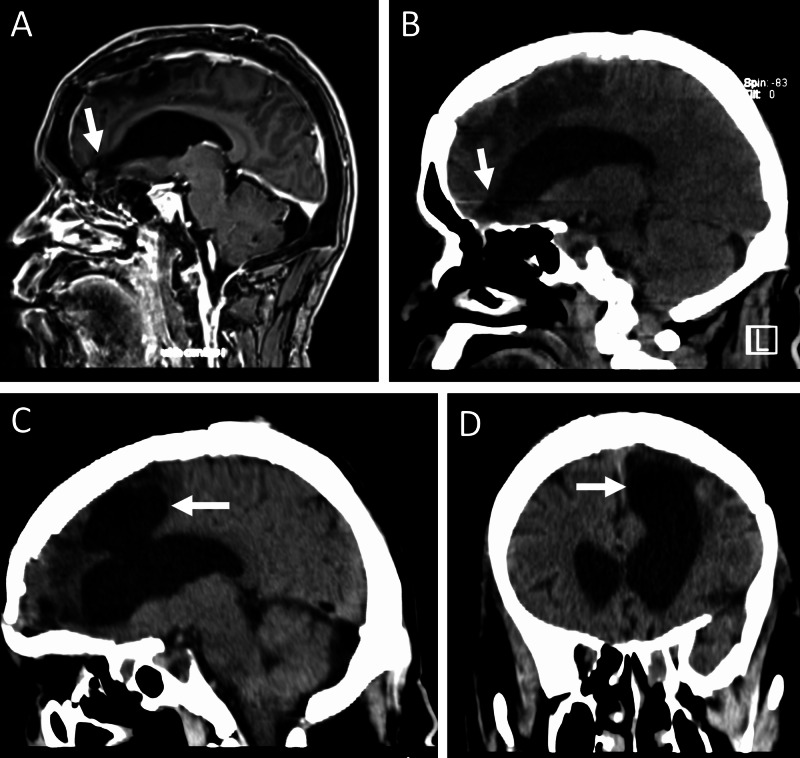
(A) MRI and (B) CT showing ventricle descending into defect in cribriform plate; (C) sagittal and (D) coronal CT showing ballooning of ventricle into previous resection cavity (not seen on scans post-tumor resection). CT, computed tomography; MRI, magnetic resonance imaging
